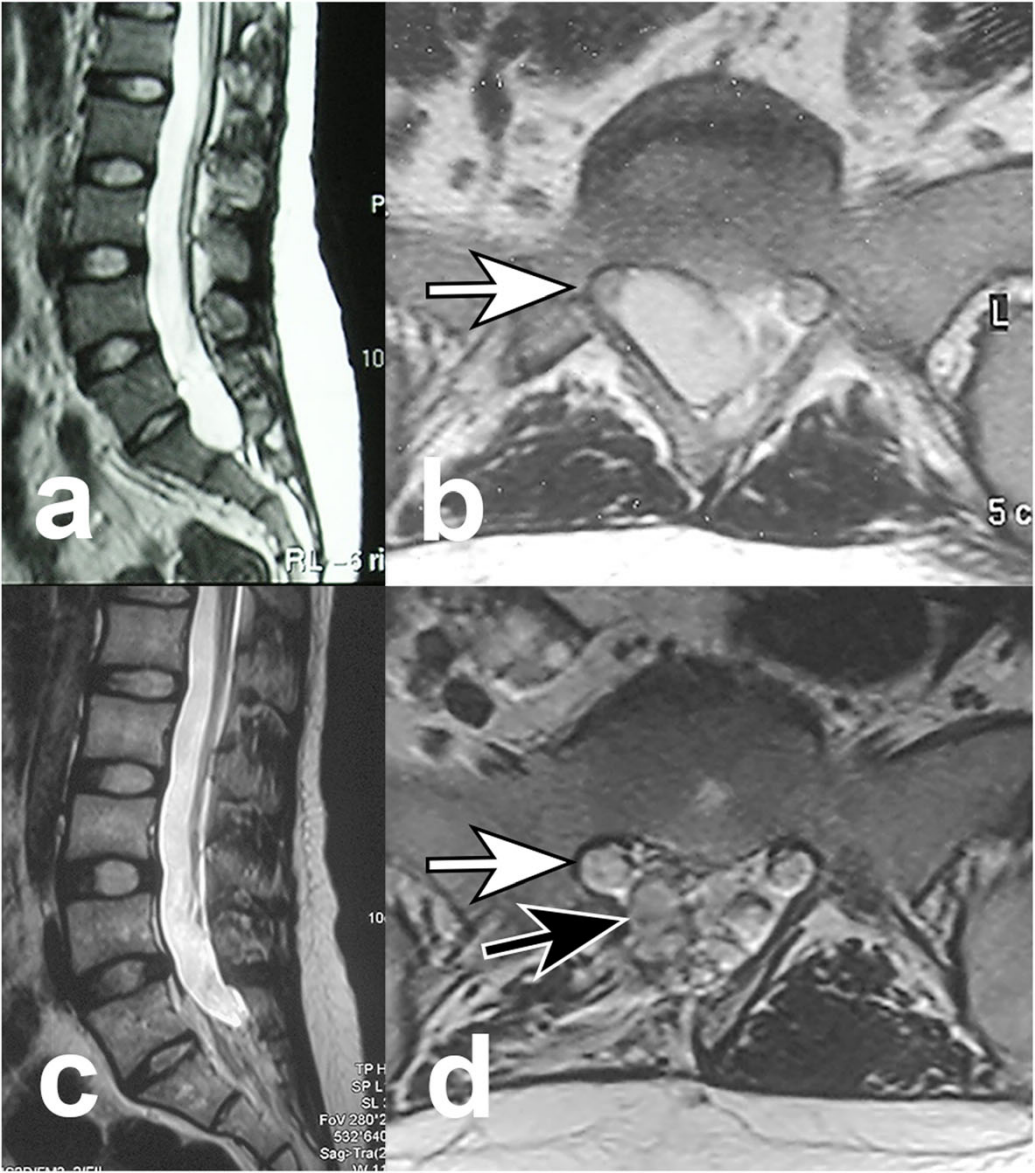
Preoperative sagittal magnetic resonance image (a) shows a sacral meningeal cyst at S1–2 level, and transverse image (b) shows cystic lesion compressing the right S1 nerve root (white arrow); Taken 64 months after surgery, sagittal image (c) shows obliteration of the cyst, transverse image (d) shows decompressed right S1 nerve root (white arrow) and stable muscle graft (black arrow)
